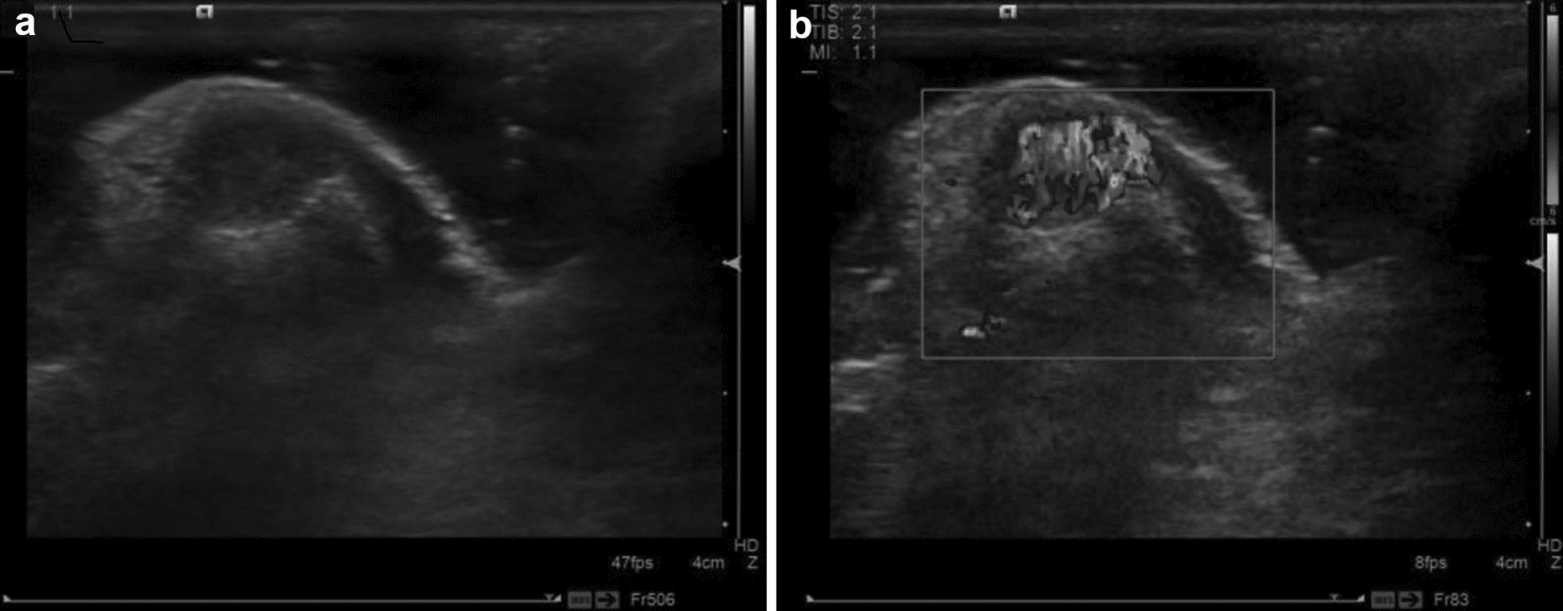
Ultrasound images of female patient (27 years old). a Two-dimensional ultrasound showed hypoechoic partial ulnar side in right thumb, with clear border and regular shape, the rear phalanx pressed to be curved; b CDFI showed rich blood flow in tumor tissues
